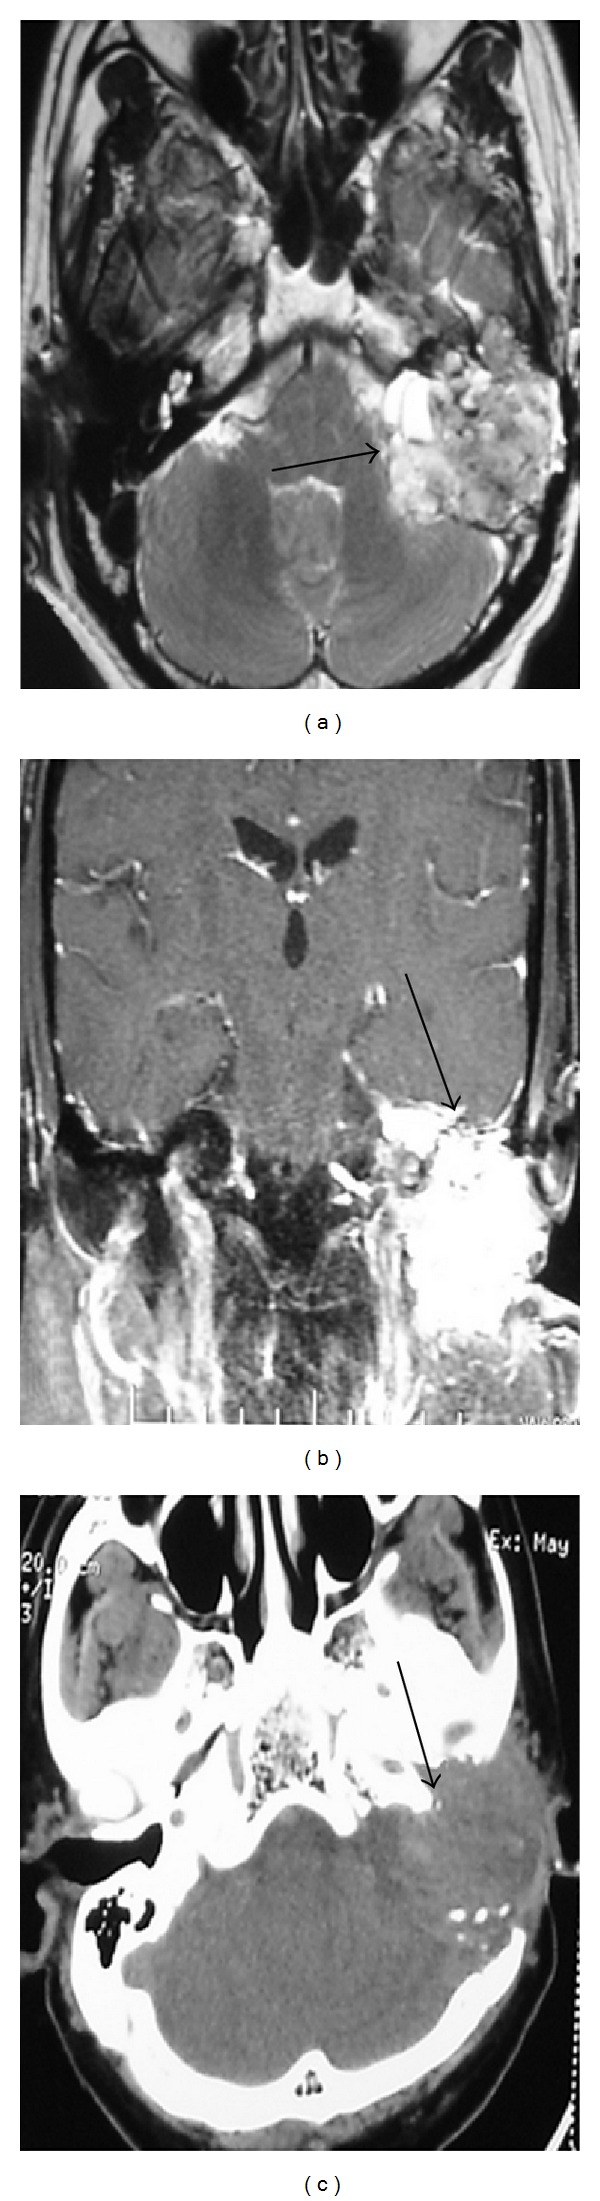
Axial MRI T2-weighted image (a) and contrast-enhanced coronal T-weighted image (b) showing heterogenous intensely enhancing mass involving left petrous temporal bone causing destruction of bone, extending medially left cerebellopontine angle cistern and inferiorly below base of skull. Axial CT scan (c) showing destruction of extensive destruction of the posterior petrous bone and middle ear structures.
